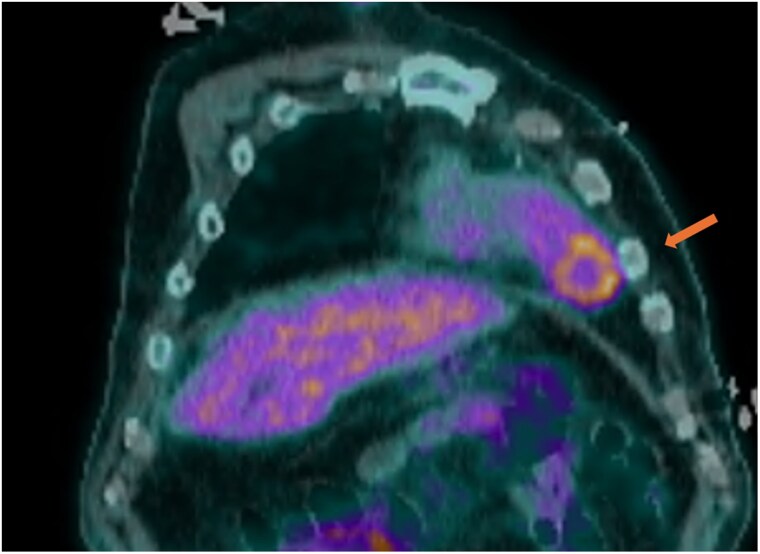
Fused coronal PET/CT image demonstrating a circular FDG-avid rim at the apex, representing peripheral inflammatory activity around an infected thrombus.
